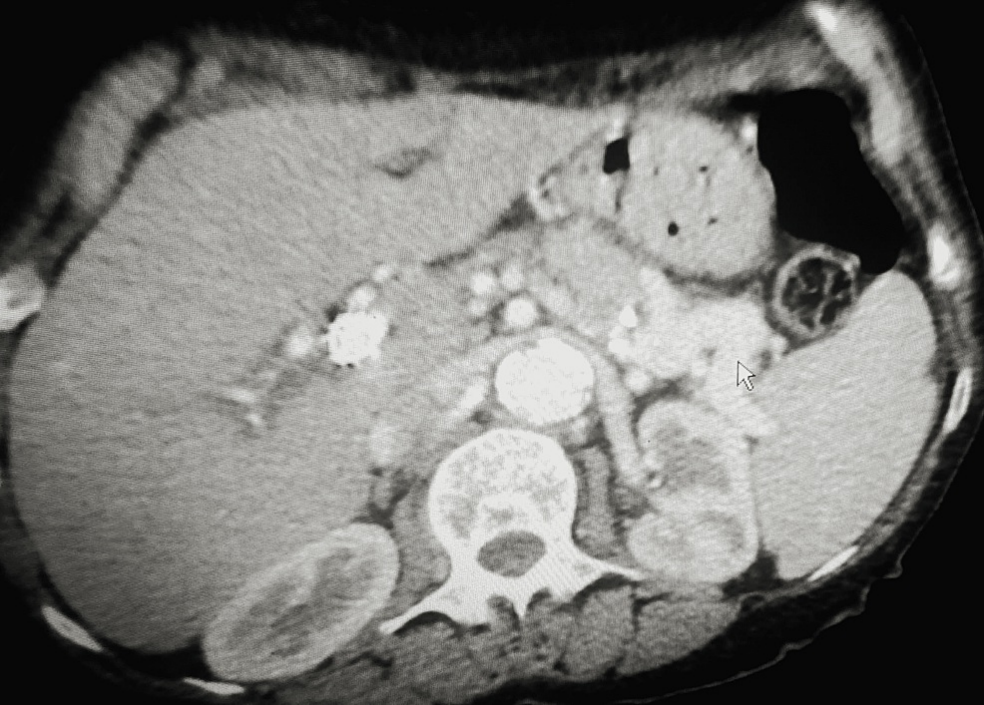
Contrast-enhanced computed tomography (CECT) of the abdomen showing dilated splenic vein (white arrow)
